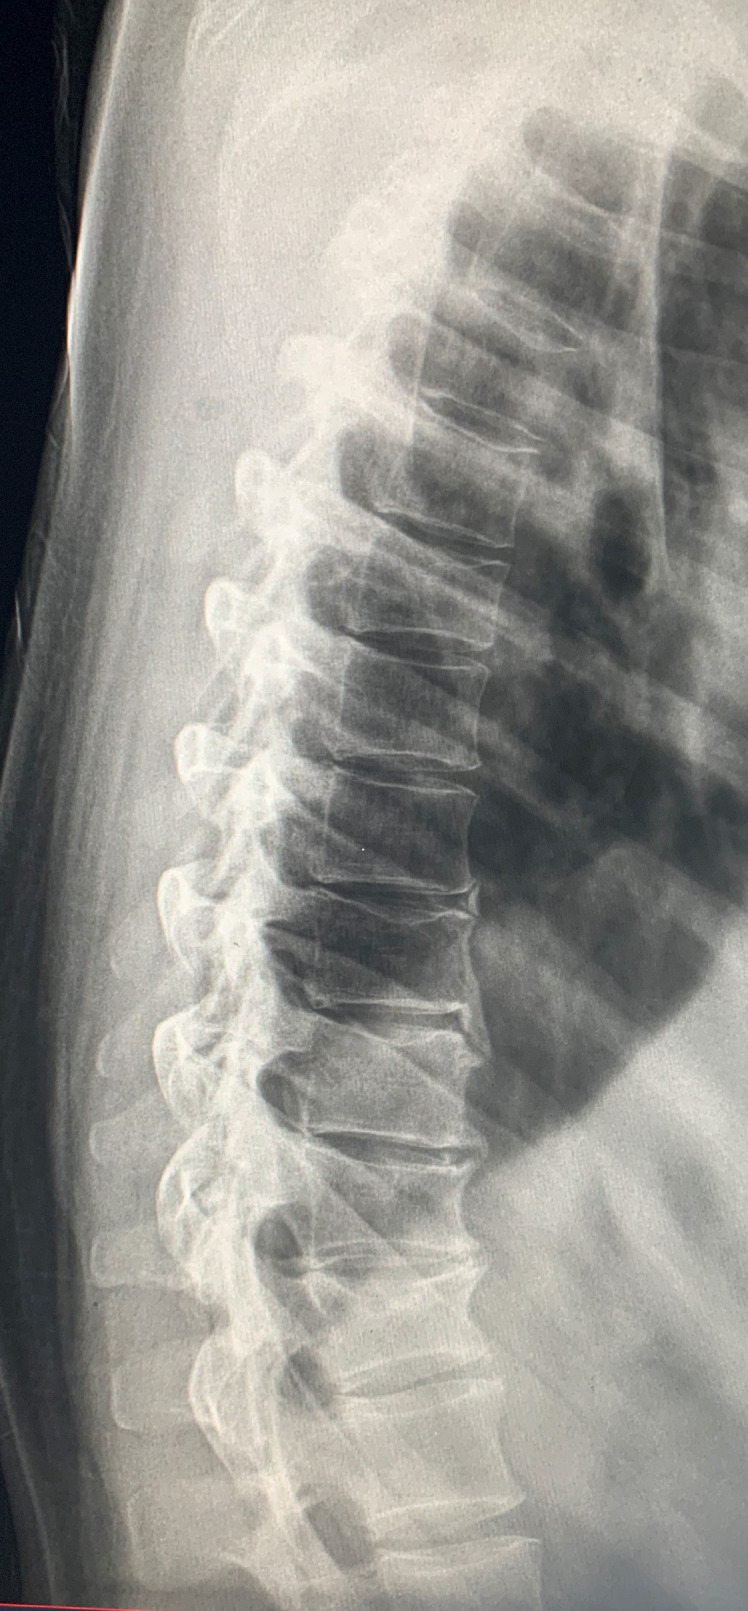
Lateral thoracic spine radiograph with multiple mild vertebral insufficiency compression fractures of T4, T5, T6,T9, T11 and moderate compression fracture of T12.
